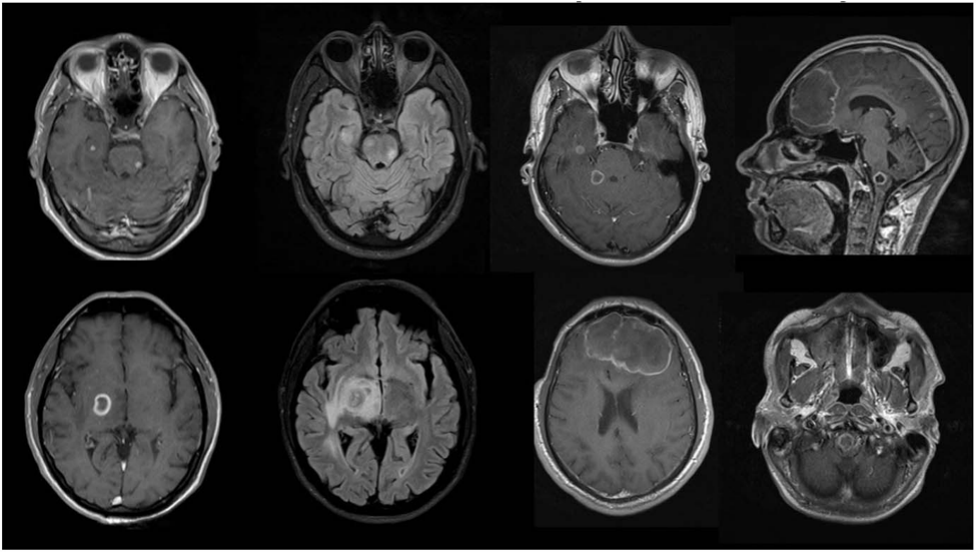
Contrast-Enhanced Brain MRI in Neuroparacoccidioidomycosis Axial and sagittal post-contrast T1-weighted MRI demonstrates round, peripheral ring-enhancing lesions with central low signal (A–C) and corresponding FLAIR hyperintensity indicating vasogenic edema (D–F). Note solitary lesions in the basal ganglia (A, D) and multiple lesions in the frontal lobe (B, E), all showing restricted diffusion on DWI (not shown). These imaging hallmarks should prompt consideration of NPCM in endemic settings.
